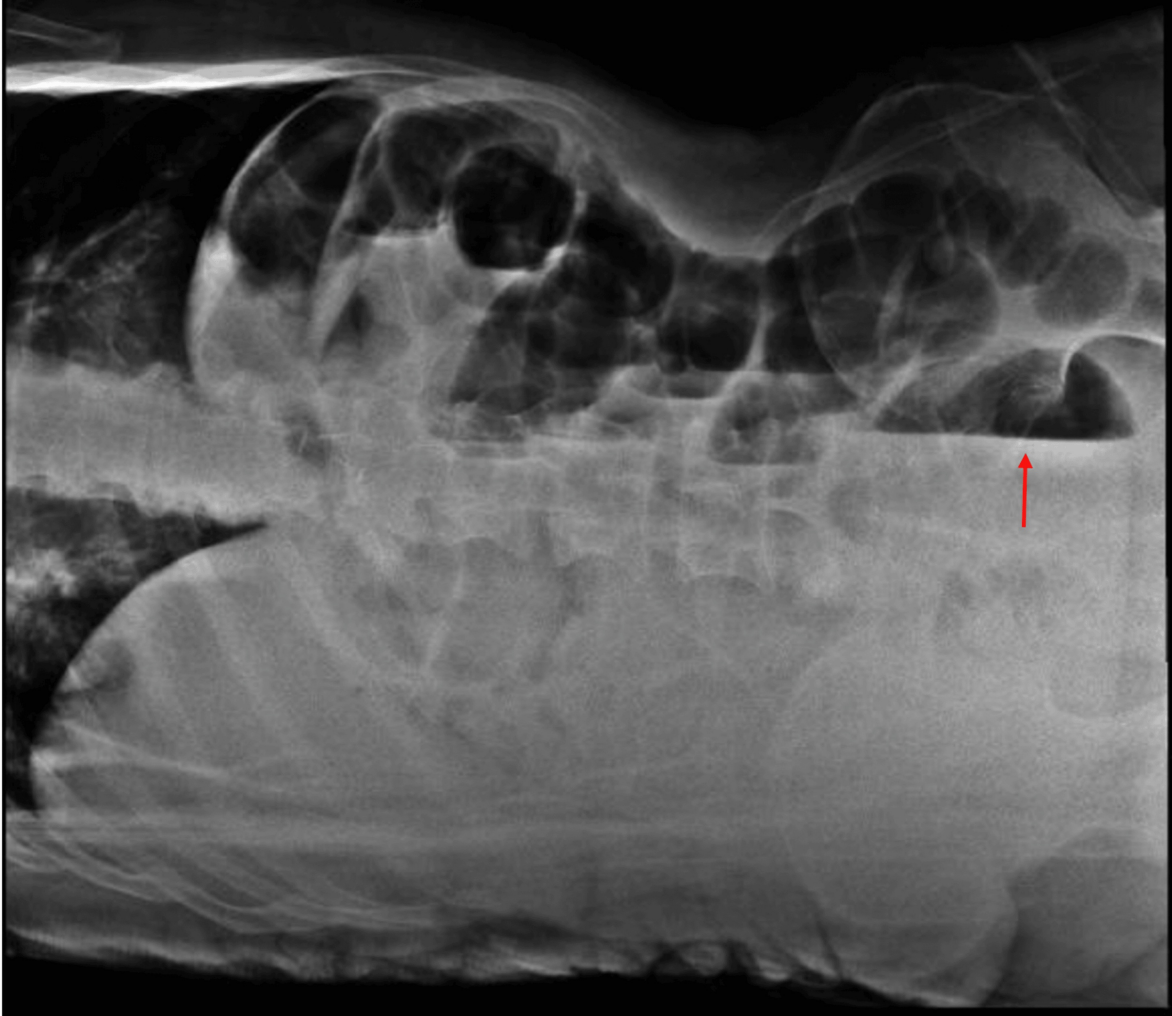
Abdominal X-ray showing air fluid levels at different levels (red arrow showing air fluid level)
